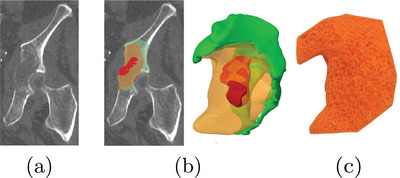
(a) Computed tomography slice of the patient's diseased wing. (b) Different areas identified on the patient's images and the CAD volume of the insert. The green area indicates the healthy trabecular bone, the orange color indicates the area with partial osteolysis secondary to metastasis, and the red color indicates the area with complete osteolysis by the metastasis. (c) Front view of the 3D‐printed insert. CAD, computer‐aided design.
